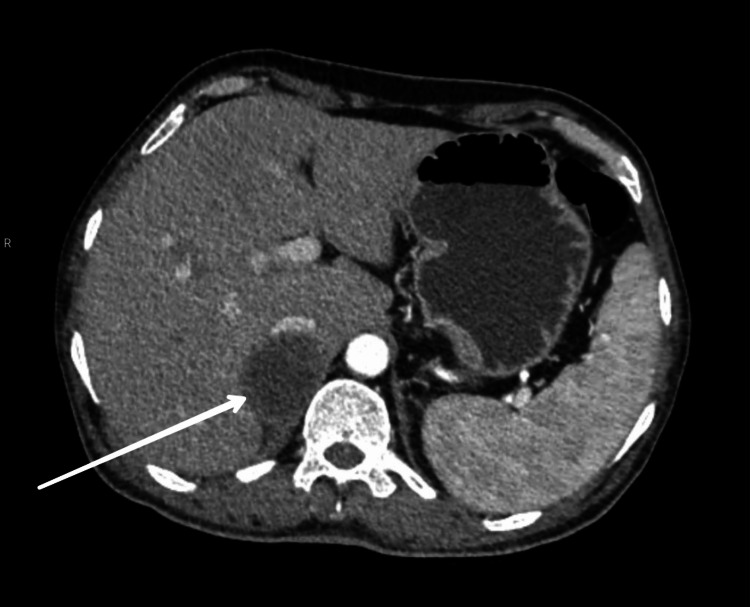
Axial contrast-enhanced CT image of the abdomen demonstrating a large, heterogeneous, soft-tissue-density right suprarenal mass (~6.3 × 4.3 × 9.3 cm; white arrow) infiltrating the adjacent liver parenchyma
